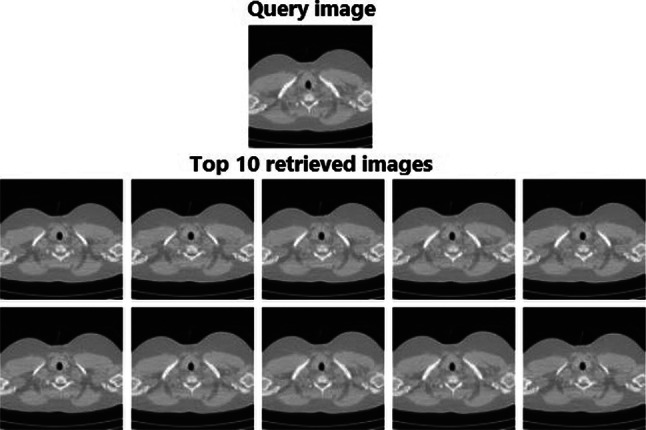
Retrieved images for an EXACT09-CT database query using RbQE with AlexNet
